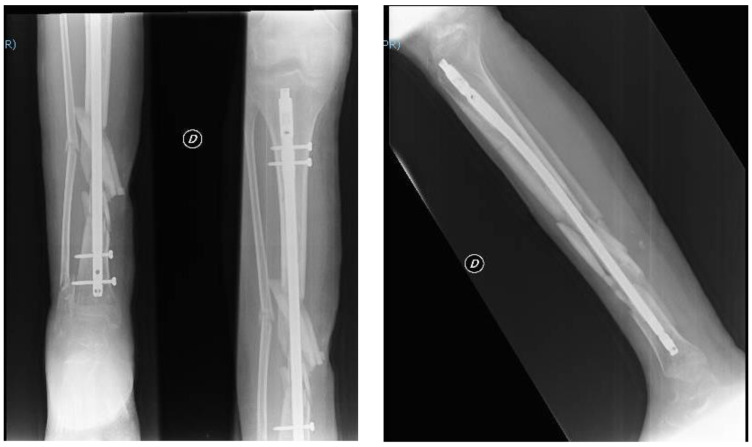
Postoperative radiograph of the right leg performed in December 2016.
